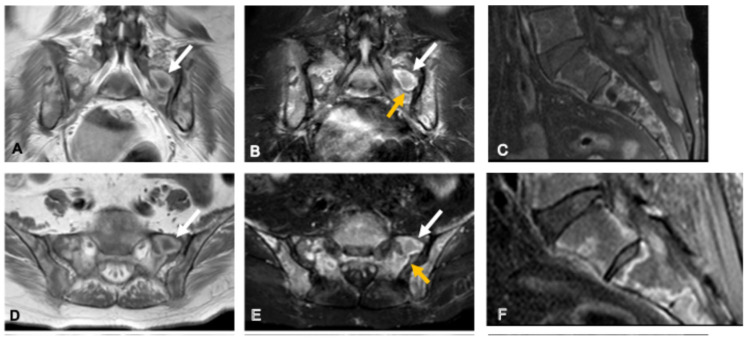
Bone marrow necrosis in a 56-year-old woman with acute lymphoblastic leukemia. Coronal T1-weighted (A) and fat-suppressed T2-weighted (B) images, sagittal contrast-enhanced fat-suppressed T1-weighted (C), axial T1-weighted (D), fat-suppressed T2-weighted (E), and sagittal contrast-enhanced fat-suppressed T1-weighted (F) sequences show extensive signal abnormalities involving the sacrum and iliac bones. The lesions exhibit areas of low signal intensity surrounded by a serpiginous rim of high signal intensity on both T1- and T2-weighted images (white arrows), producing a geographic pattern. Some lesions demonstrate an additional outer rim of low signal intensity on T2-weighted images (yellow arrows). Post-contrast images (C,F) reveal peripheral enhancement corresponding to reactive granulation tissue. The overall imaging appearance is atypical for lymphomatous infiltration and more consistent with bone infarction. CT-guided biopsy confirmed bone marrow necrosis, identified at leukemia diagnosis prior to treatment initiation.
